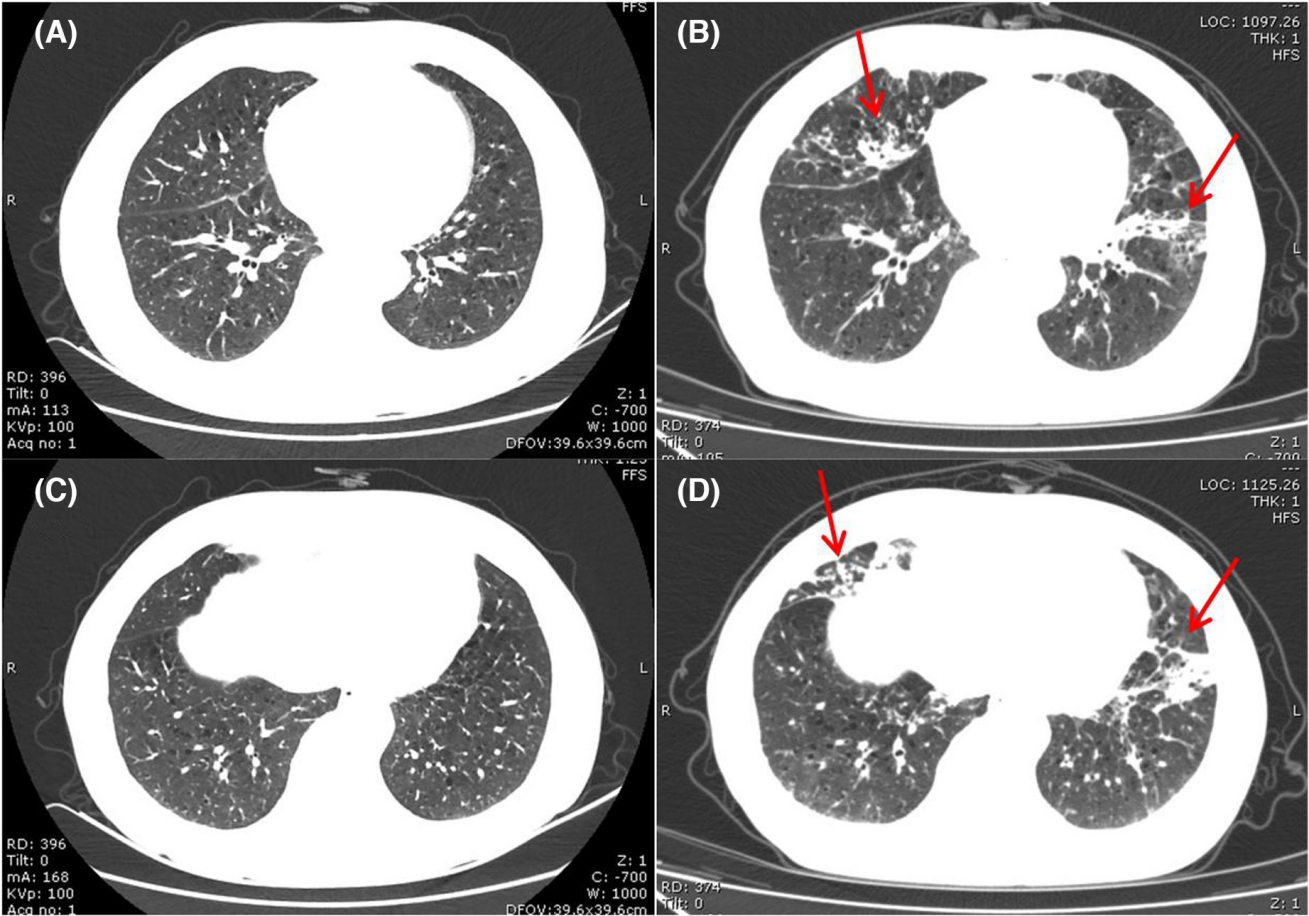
OP pattern in a 69‐year‐old man who underwent Keytruda therapy for stage IV lung Squamous cell carcinoma. (A) and (C) A baseline chest CT image before starting immunotherapy. (B) and (D) Axial chest CT image obtained 3 months after starting immune checkpoint inhibitor therapy shows multifocal subpleural mid‐ and lower‐lung consolidations (arrows).
